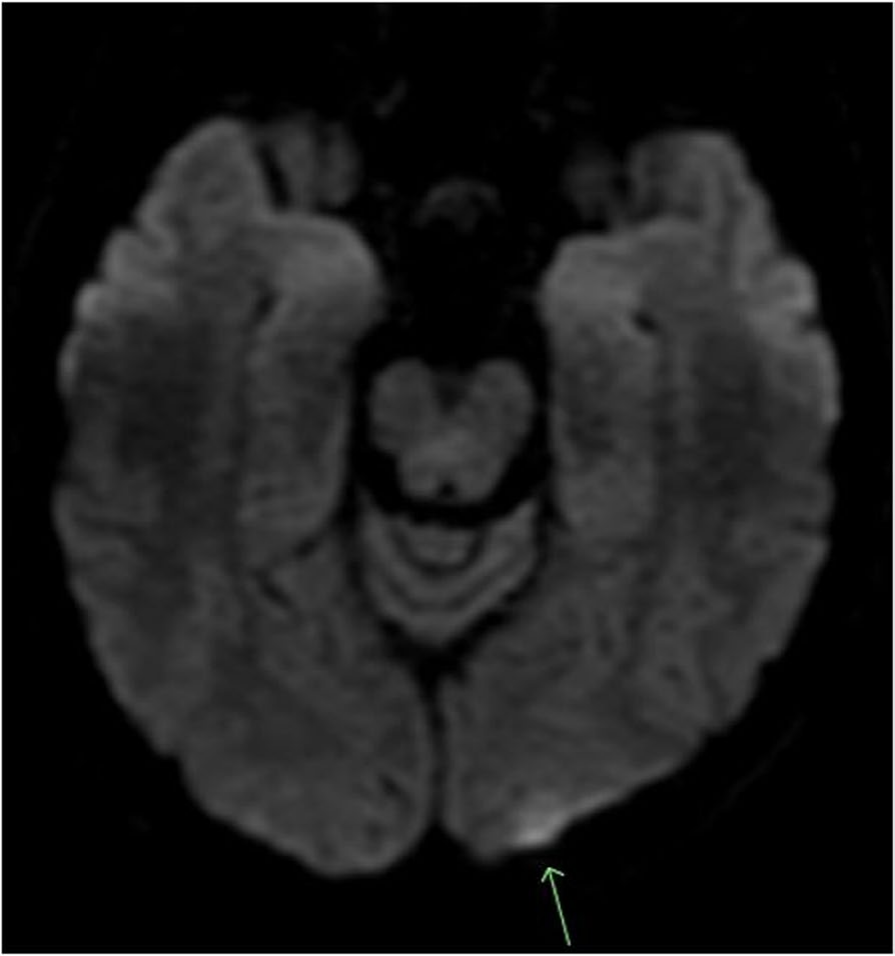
MRI Brain DWI revealing left cortical hyperintensity consistent with acute infarct at watershed zone
